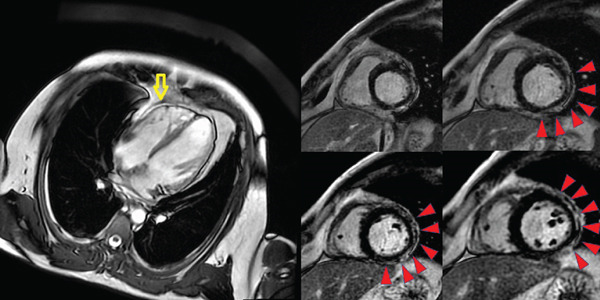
Cardiac magnetic resonance imaging. The yellow arrow indicates mild RV dilation and trabeculation with apical hypokinesia. The red arrow shows subepicardial LGE in the anterolateral and lateral LV segments, consistent with arrhythmogenic cardiomyopathy.
